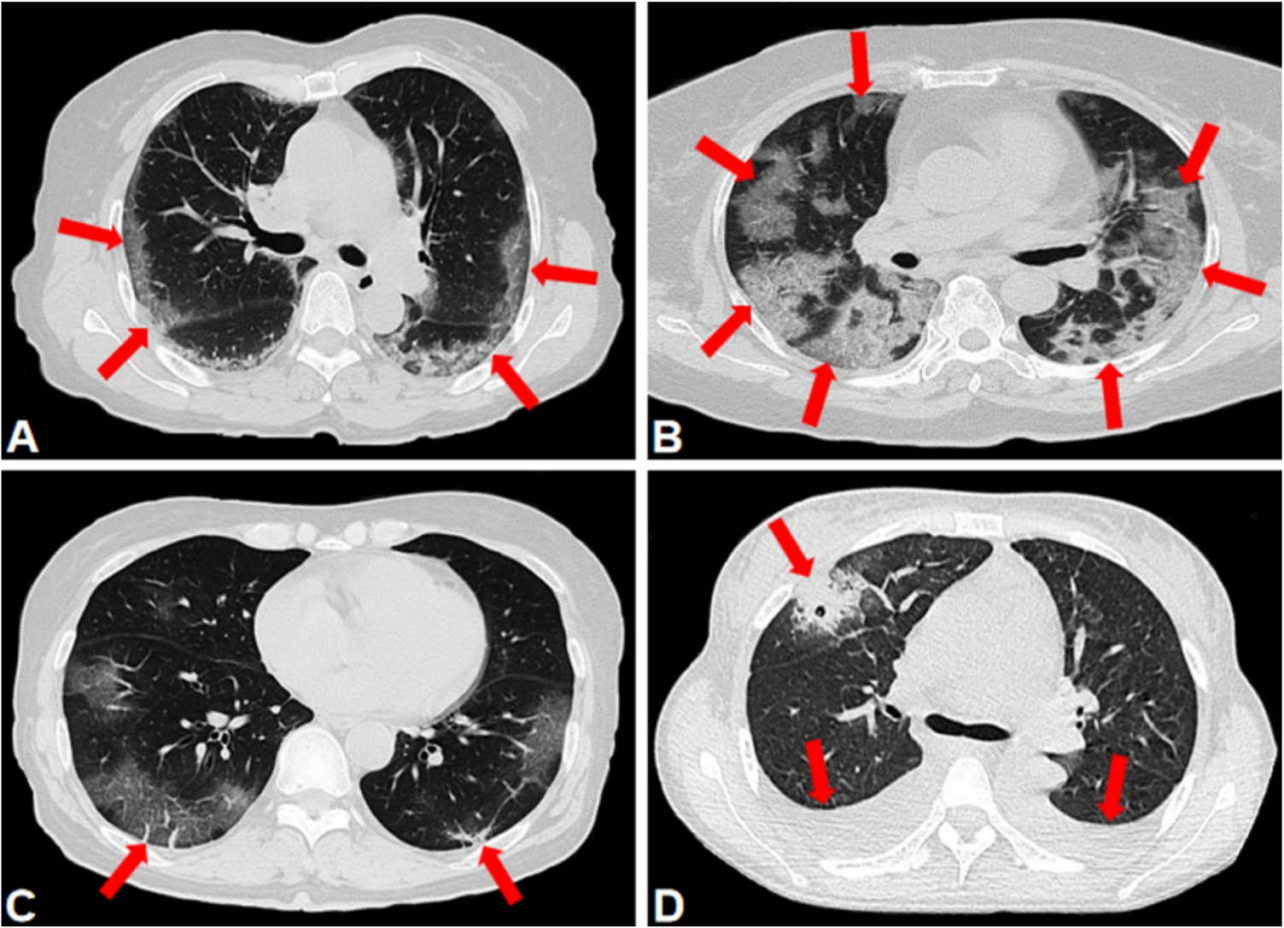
Chest CT features of COVID-19. A Male, 65 years, confirmed as COVID-19, chest CT images showed crazy-paving pattern and interstitial change (arrows); B Male, 74 years, confirmed as COVID-19, chest CT images showed GGO with consolidation, crazy-paving pattern, multiple, and irregular shape (arrows); C Male, 68 years, confirmed as COVID-19, chest CT images showed GGO and interstitial change (arrows); D Male, 59 years, confirmed as COVID-19, chest CT images showed GGO with consolidation and pleural effusion (arrows)
